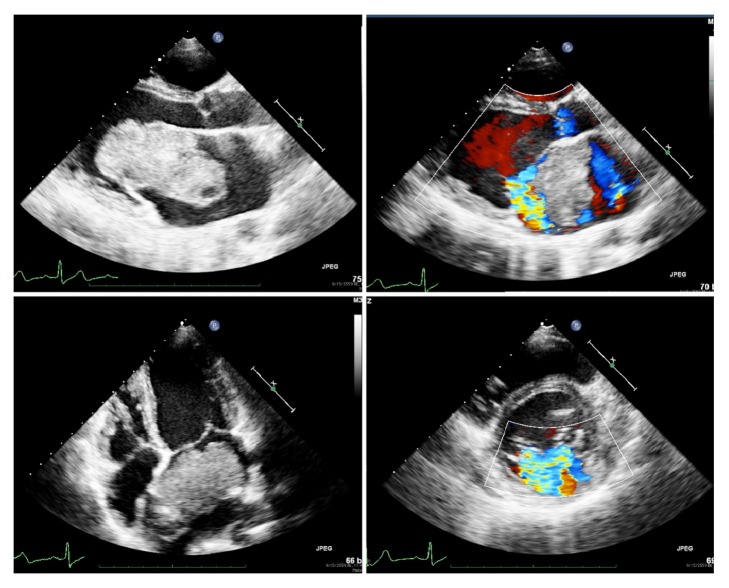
Preoperative echocardiography showed huge left atrial mass protruding through mitral valve with severe mitral insufficiency.
